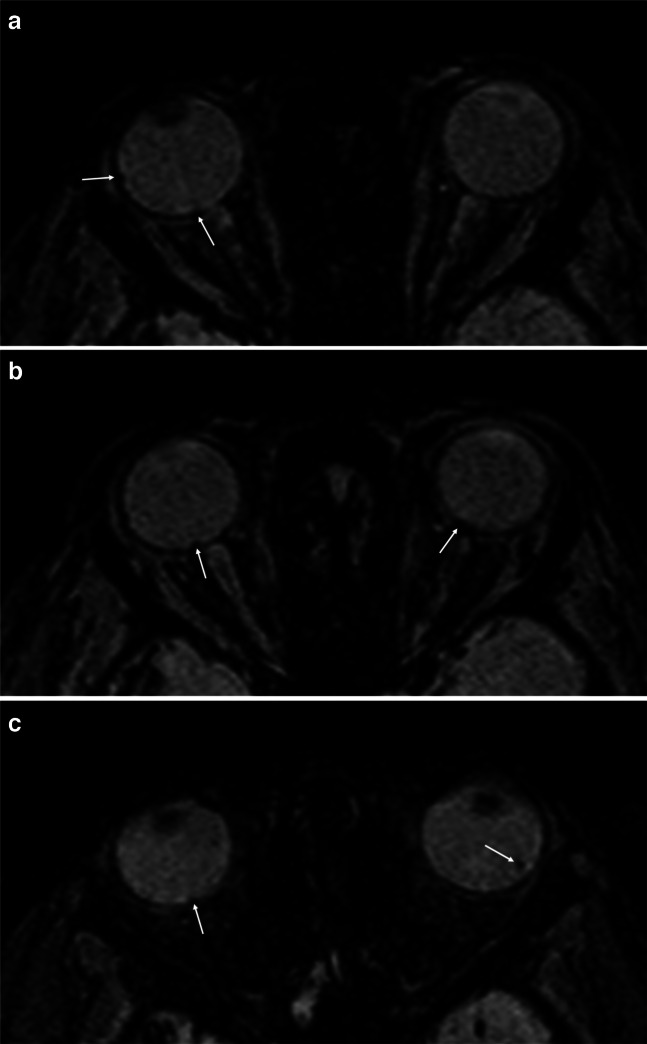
An 8-month-old boy with abusive head trauma following shaking and right-sided retinal hemorrhages detected on fundoscopy. In the right eye, pronounced streak-like epi- and subretinal hemorrhages were observed. The left eye showed central retinal edema without evidence of hemorrhage. a, b, c Axial susceptibility-weighted magnetic resonance images through different orbital planes demonstrate small signal voids within the retina at the posterior poles of both eyes and additionally at the lateral poles of both eyes (*arrows*)
